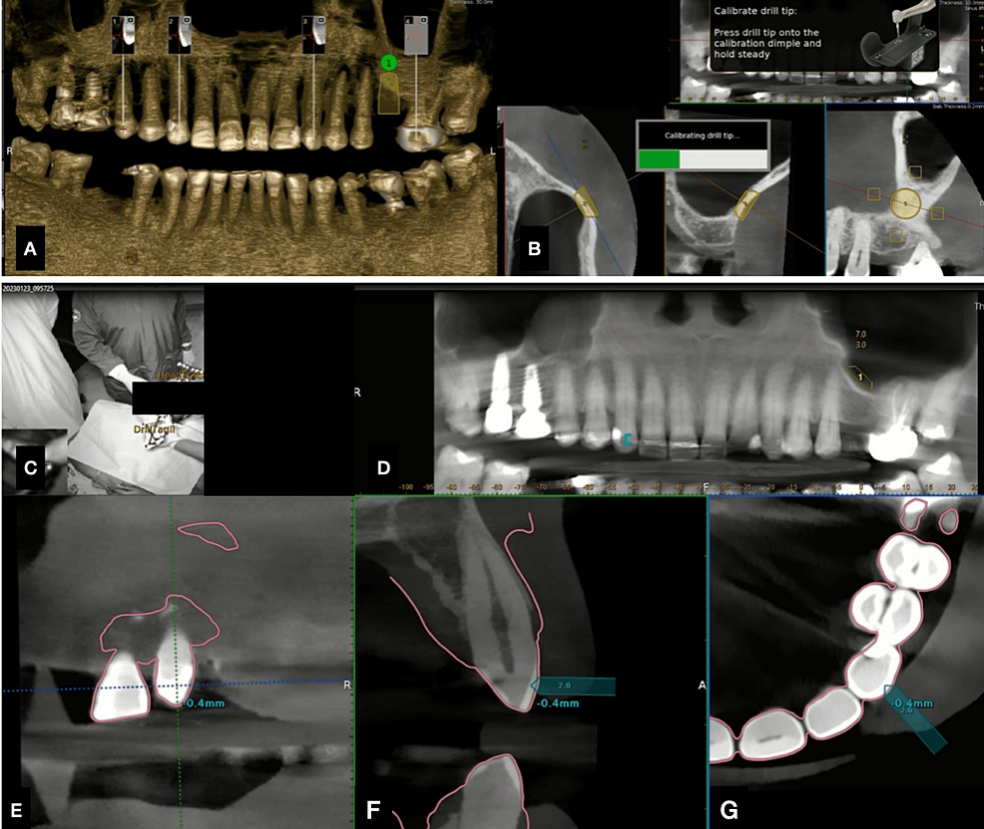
Working with dynamic navigation (A) Points in the cone beam computed tomography (CBCT) for trace registration. (B) Calibration of the drill tip. (C) Drill tag and jaw tracker detected by the computer. (D) Accuracy checking of the trace registration using the drill tip on the cusp of the index tooth. (E) Accuracy checking with the drill tip. (F) Accuracy check with the drill tip of the adjacent tooth. (G) Occlusal region accuracy check of the calibrated data
